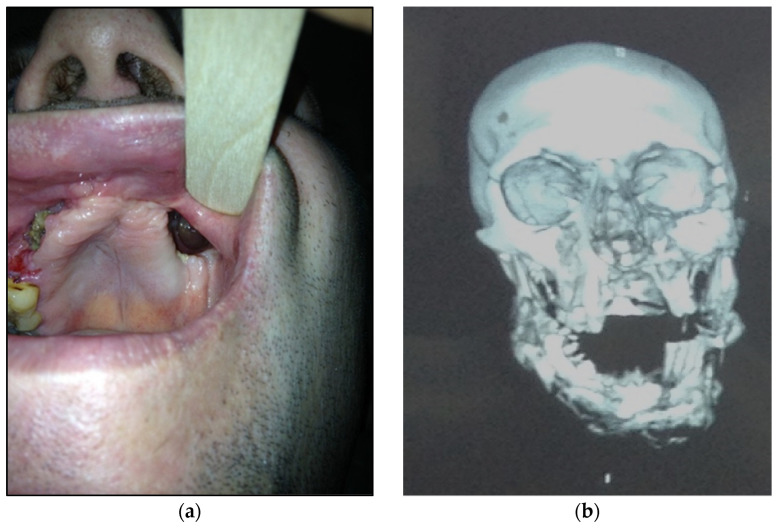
(a) Patient with type 4 toxic phosphorus osteonecrosis. Intraoral view; (b) multi CT-scan of patient’s skull before operation.
